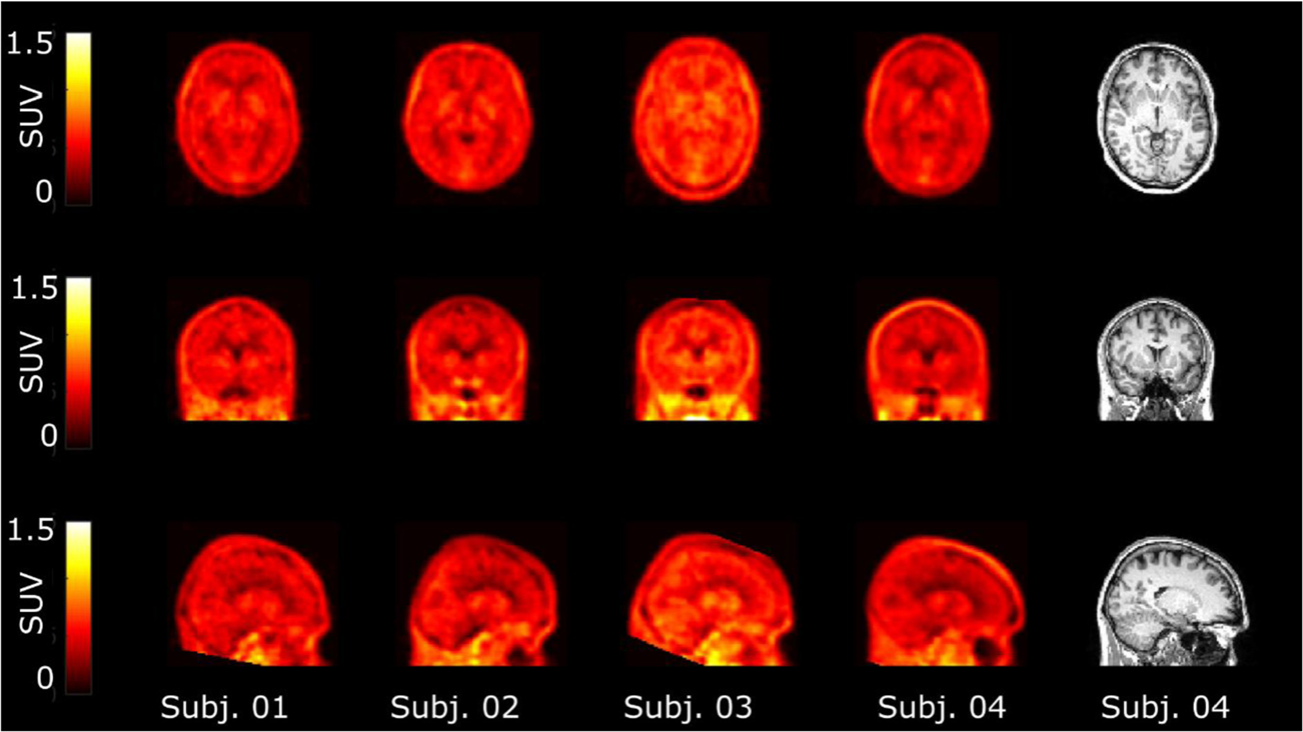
Images showing the summed radioactivity (60–90 min) in the brain of [^18^F]GSK2647544 (*left four panels*) with T1 weighted structural MRI scan show for subject 4 (*right panel*). All four subjects received a 100 mg of unlabelled GSK2647544 followed 2 h later by a bolus injection of [^18^F]GSK2647544 (injected dose: 106 ± 47 MBq). The uptake is expressed standardised uptake values (SUV).
